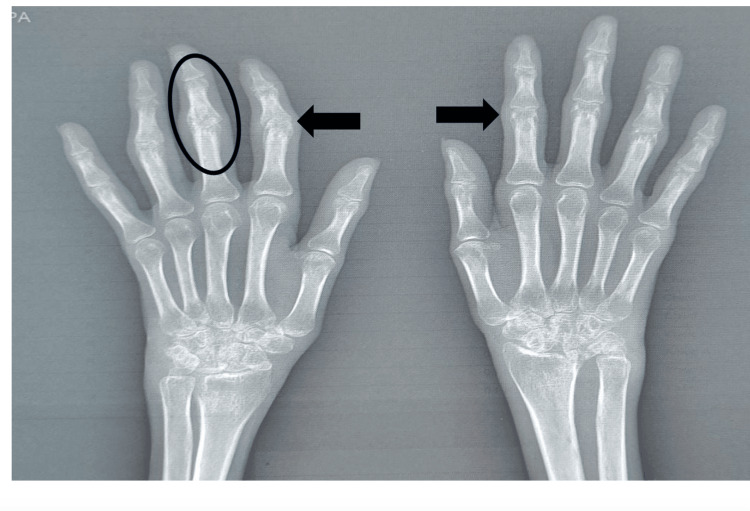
X-ray images of both hands The image shows reduced joint space, subchondral osteopenia, articular marginal erosions in proximal interphalangeal joints bilaterally, and ankylosis of the third PIP joint of the right hand.
